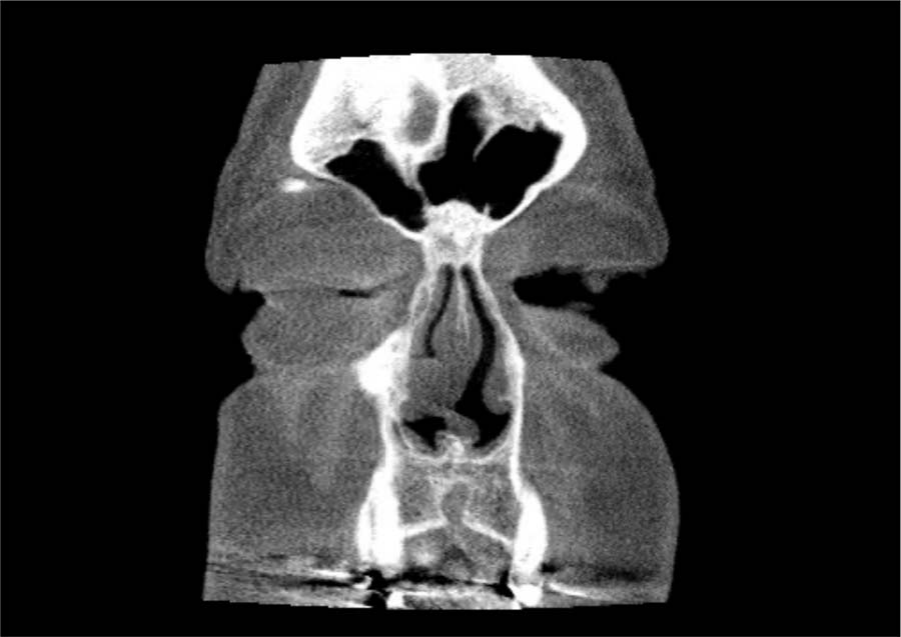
Coronal non-contrast CT scan images of soft tissue lesion on right inferior turbinate.
